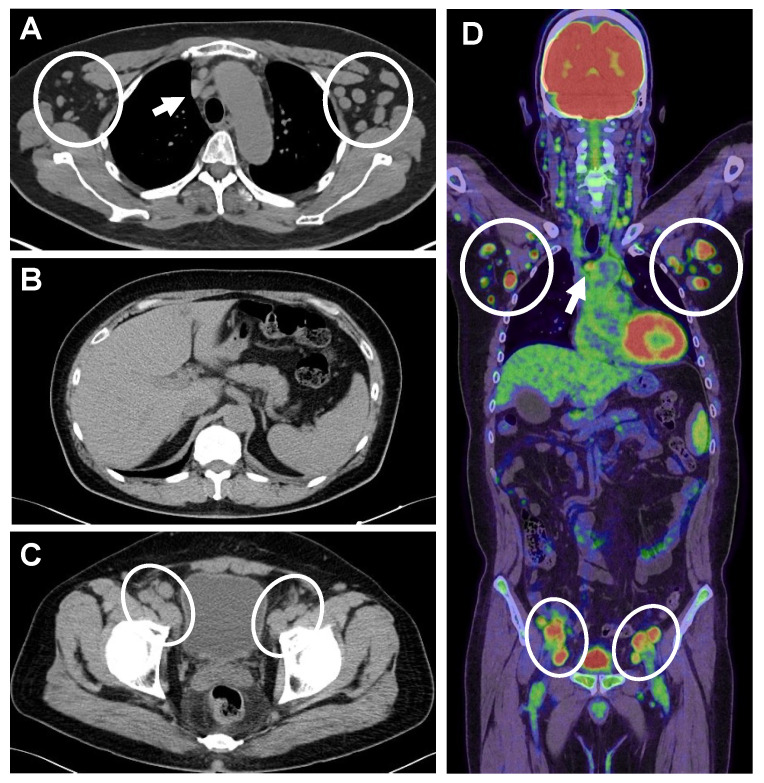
PET-CT imaging. (A) Enlargement of axillary (circles) and mediastinal lymph nodes (arrow). (B) No hepatosplenomegaly or ascites are seen. (C) Inguinal lymph node enlargement (circle). (D) FDG accumulation consistent with enlarged lymph nodes (circles).
